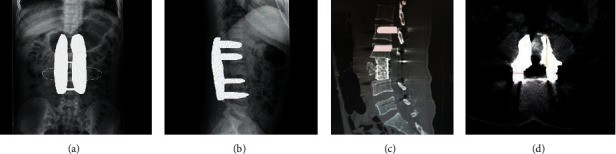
Conventional CT scan images. (a) Coronal position of lumbar vertebra. (b) Sagittal position of lumbar vertebra. (c) Sagittal position of thoracic vertebra. (d) Transverse position of lumbar vertebra. Many metal artifacts were observed, and the relationship among artificial joints and the position of internal fixation nails were blurred.
